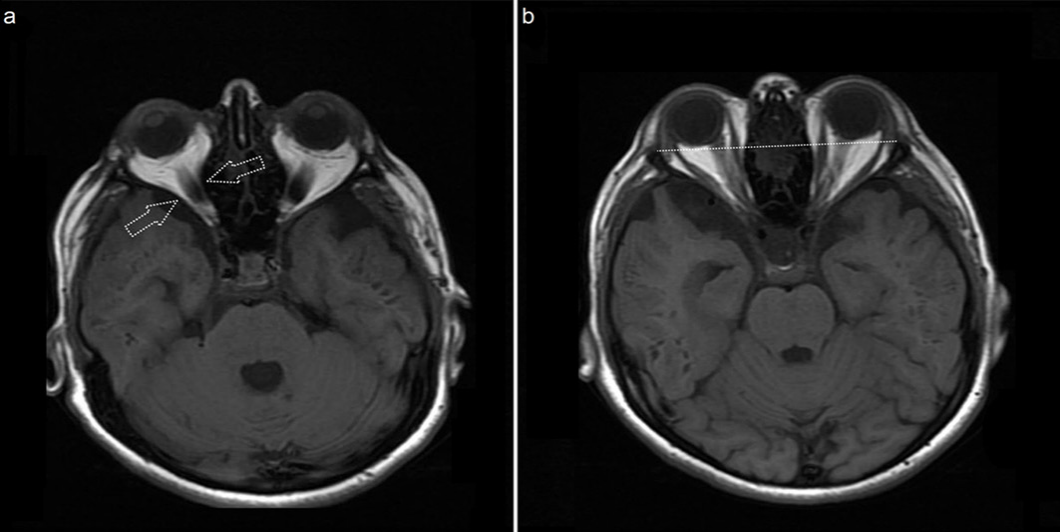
Axial T1 weighted MR image (a) depicts that the orbital apex is narrowed bilaterally and formed as a sandglass appearance (white arrows). Axial T1 weighted MR image (b) reveals bilateral exophthalmos (white dotted line) (15 years old, MPS type II).
